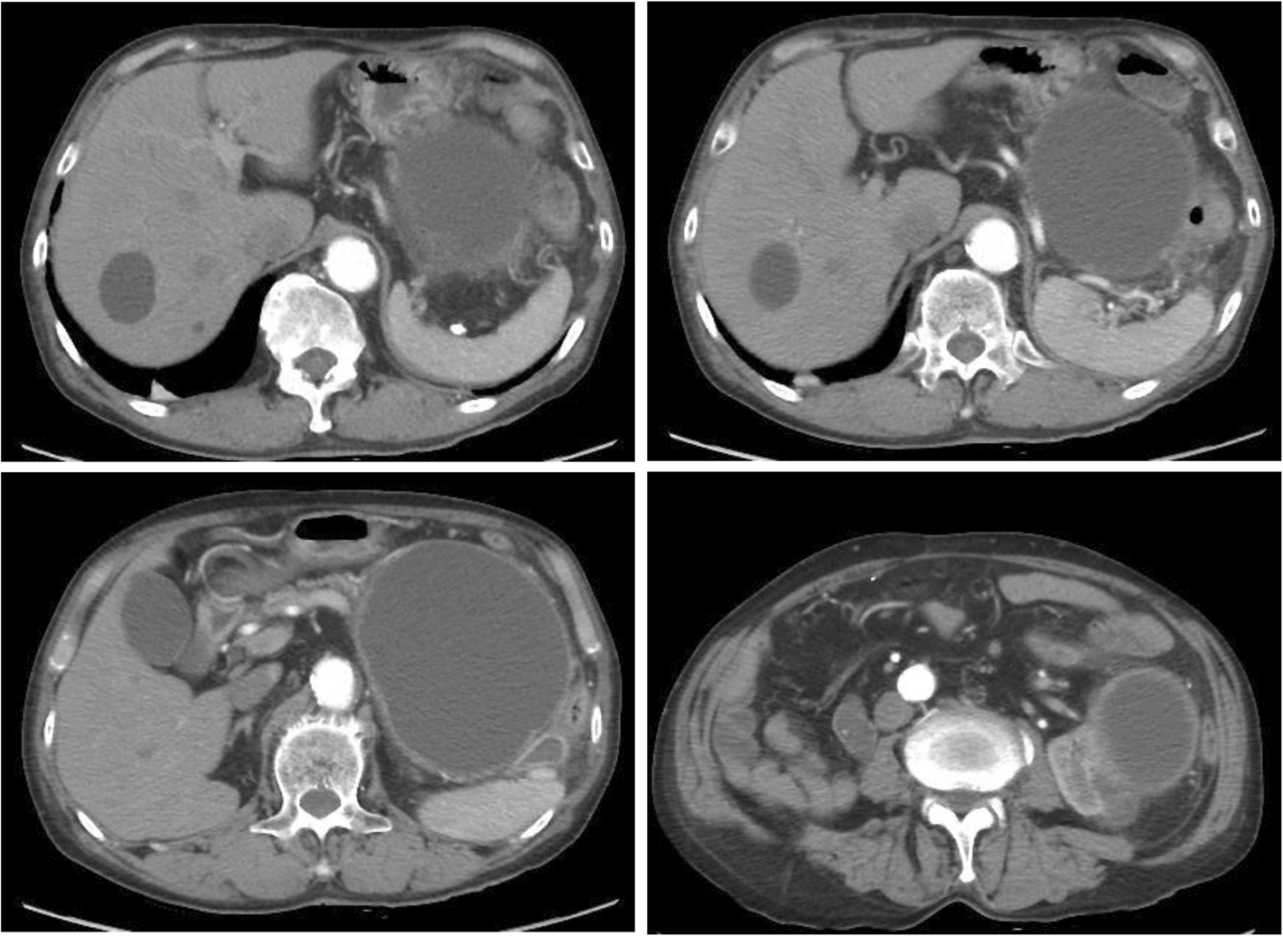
Computed tomography (CT) findings. Enhanced CT revealed a cystic lesion in the body of the pancreas measuring 119 mm × 100 mm and an adjacent left renal cyst of 63 mm in diameter. The pancreas cyst wall was thickened. The pancreatic ducts were not dilated
